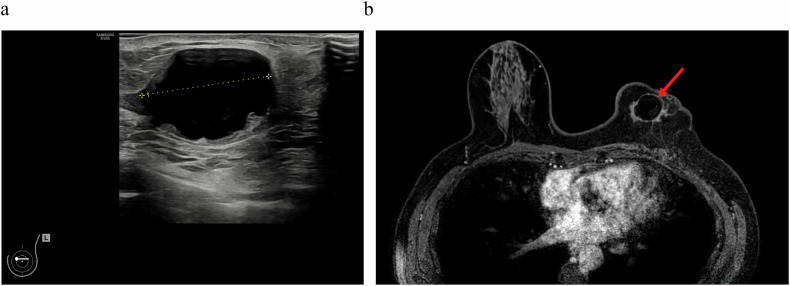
Fat necrosis after total AFT breast reconstruction. A 61-year-old patient with a unilateral mastectomy of the left breast because of DCIS, presented with a palpable lump almost 21 months after the fourth session for total AFT reconstruction. US and MRI examination showed fat necrosis. On US, multiple cysts of < 1 cm were found in the upper outer quadrant of the breast, as well as a round cyst of 2.7 cm retroareolar (a). MRI dynamic weighted images showed multiple oil cysts, with hyperintense borders (b; arrow). Both exams were classified as BI-RADS 2
